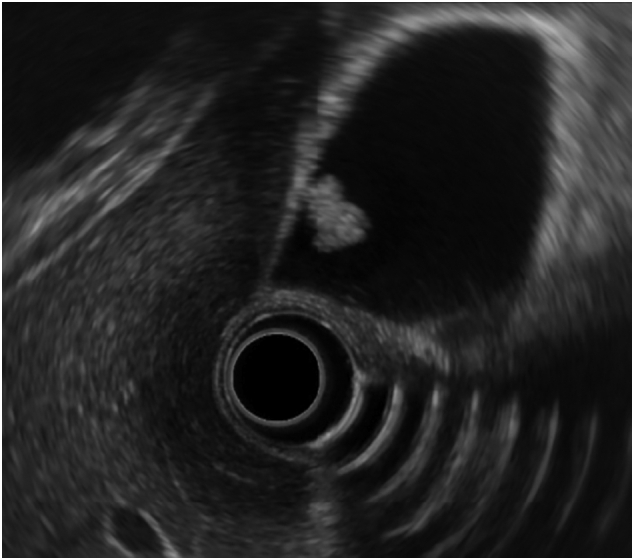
Endoscopic ultrasound (EUS) showing a hyper echoic pedunculated shaped tumor 8 mm in size located in the bottom of the gallbladder with a parenchymal-like internal echogenic pattern.
